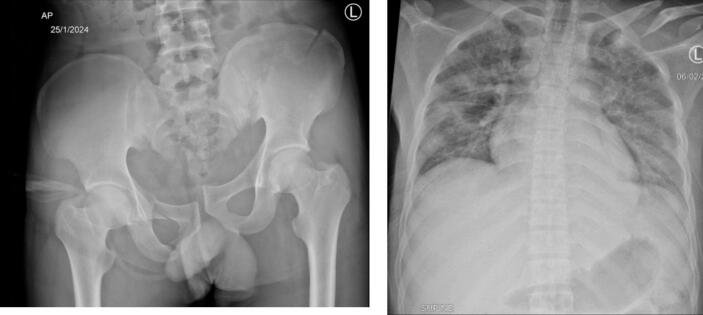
An anteroposterior (AP) pelvic radiograph, revealing a pelvic ring fracture(left) and a posteroanterior (PA) chest radiograph (right) shows a left clavicle fracture.
